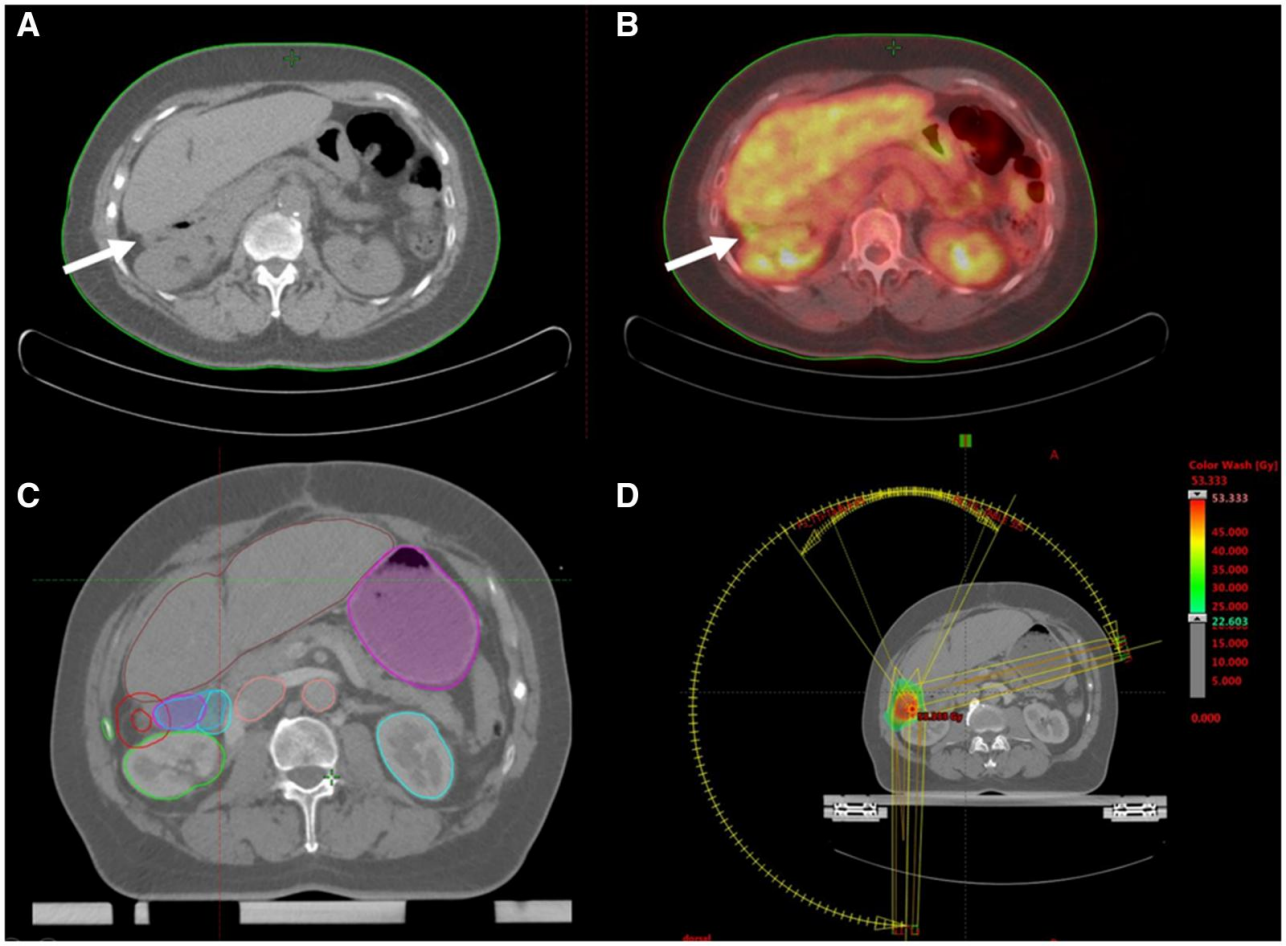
Peritoneal metastasis (right Morrison pouch) managed by SBRT: radiologic and dosimetric features. (A) Unenhanced CT image demonstrating peritoneal metastasis in the right Morrison pouch. (B) ^18^F-fluorodeoxyglucose positron emission tomography/computed tomography (PET/CT) showing uptake of the peritoneal metastasis. (C) Unenhanced CT-scan with the gross tumour volume (GTV) encompassing the lesion. The planning target volume (PTV) covered the GTV with 5 mm margin. The treatment was performed with 6 megavoltage (MV) flattening filter free (FFF) photons on a linear accelerator using VMAT technique. (D) VMAT plan showing axial images of radiotherapy dose colour wash, with a hot spot (53.333 Gy) within the PTV.
